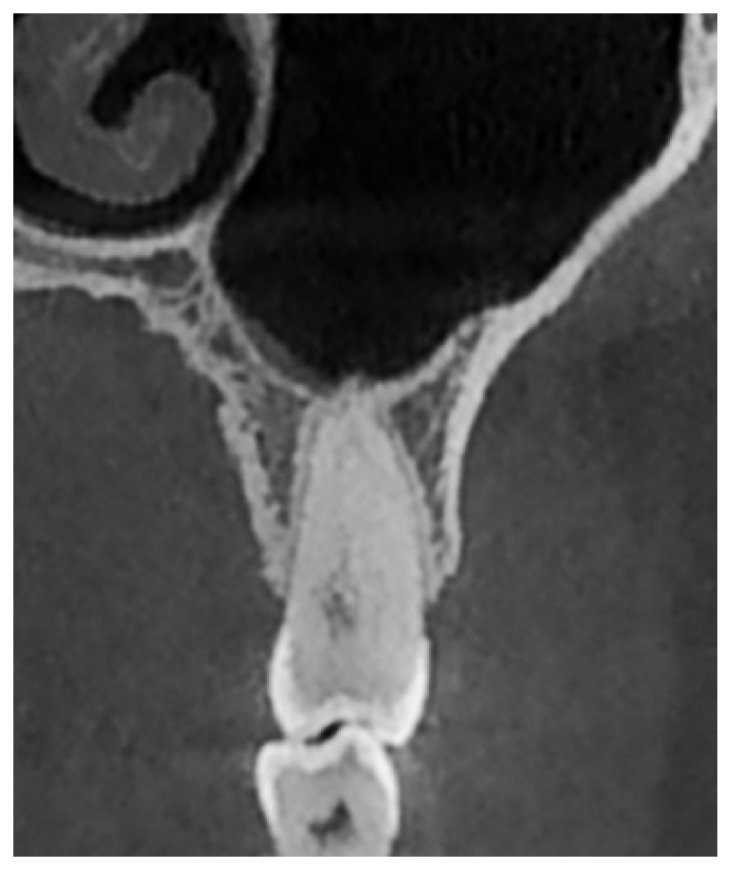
Cross-sectional CBCT reformat along the long axis of tooth 25. Tangential-type relationship between tooth 25 and the maxillary sinus.
